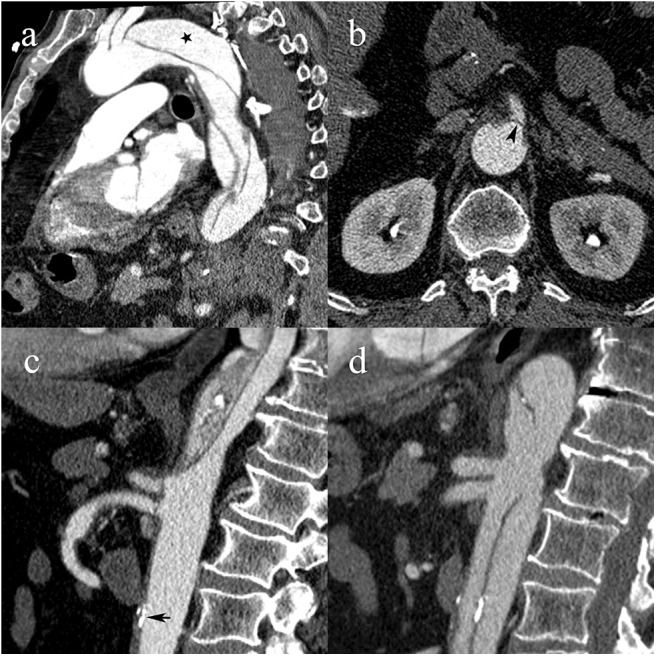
A 64-year-old male patient with type B1 AAD. Intimomedial flap arising distally to the left subclavian artery with patent false lumen (black star) (a); flap prolapsing over the ostium of the superior mesenteric artery, causing dynamic MPS (black arrowhead) (b); complete collapse of the true lumen due to hemodynamic forces draping the dissecting membrane against the anterior wall of the aorta and prolapsing the flap over the ostia of the celiac and superior mesenteric artery. Atherosclerotic calcifications are in line with the aortic wall (black arrow) (c); follow-up CTA shows patent true lumen following urgent endovascular fenestration, restoration of celiac and superior mesenteric artery blood flow, and atherosclerotic calcifications now displaced into the lumen (d).
